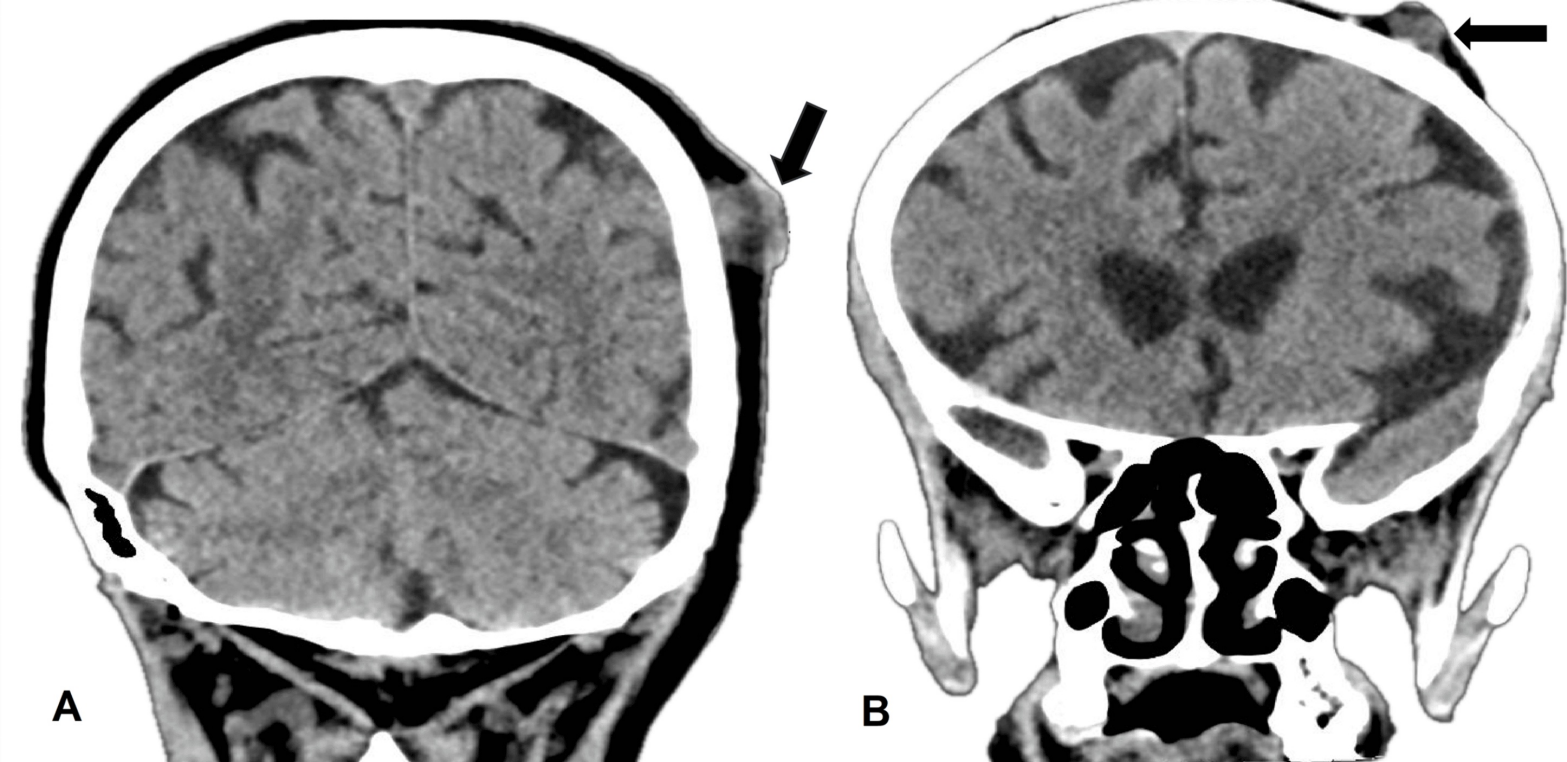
Computed tomography findings A: CT brain showing a metastatic renal cell carcinoma scalp mass in the temporal area (arrow); B: CT brain showing a metastatic renal cell carcinoma scalp mass in the frontal area (arrow)
